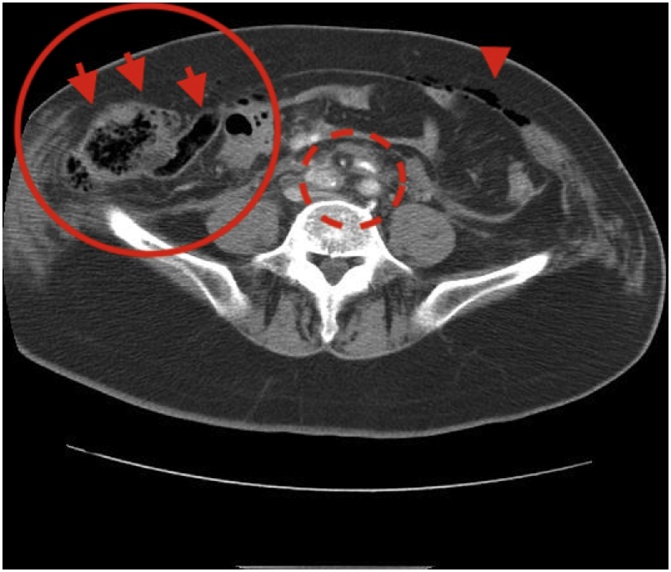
Enhanced computed tomography scan on admission.The scan revealed a defect in the abdominal wall (circle), herniation of the cecum and small bowel loops (arrow), free air in the abdomen (arrowheads), and an acute traumatic dissection of the abdominal aorta and common iliac artery (dotted circle).
